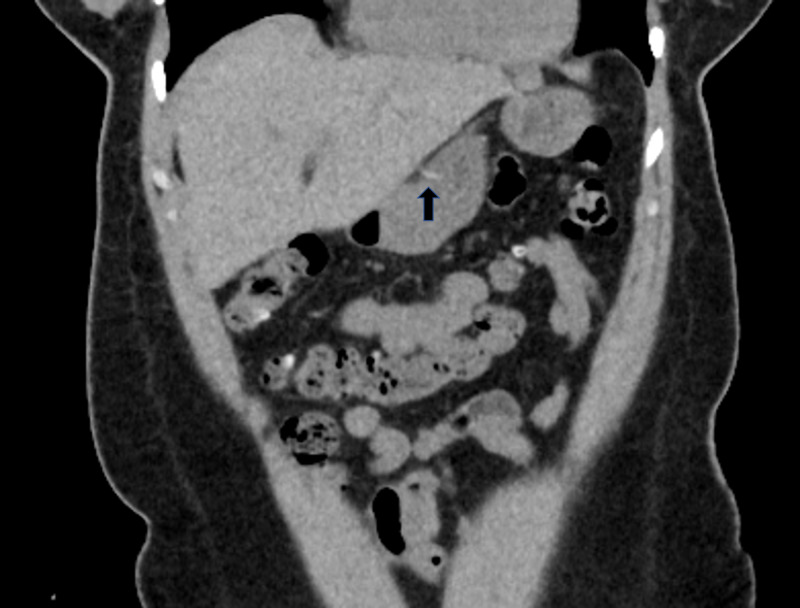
Abdominal CT scan revealing toothpick in distal body of stomach extending out of the lumen
